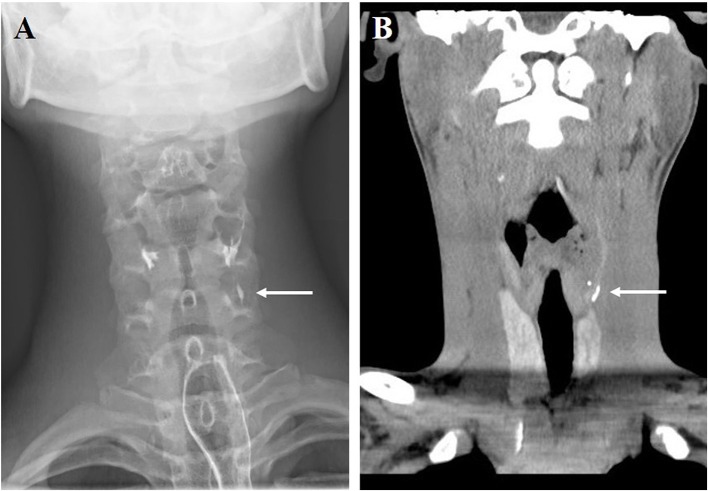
Barium esophagography (A) and CT coronal scan (B) showed pyriform sinus fistula in non-infected state (arrow, the same patient in Figure 1).
